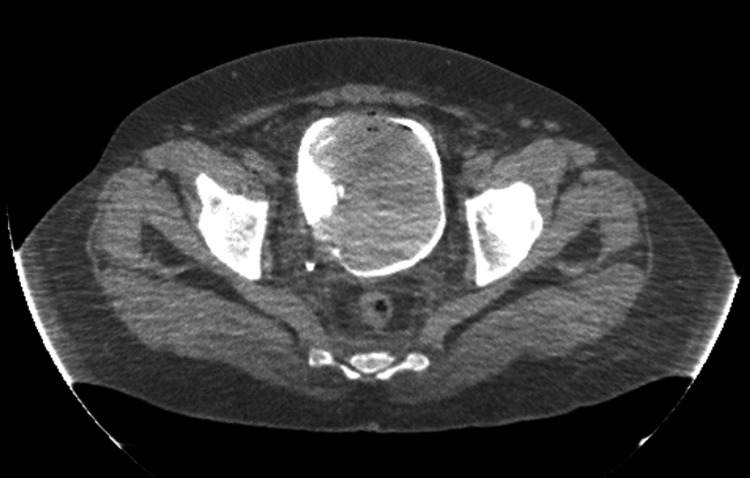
A computed tomography-based cystography showing a 10 cm bladder clot mass
